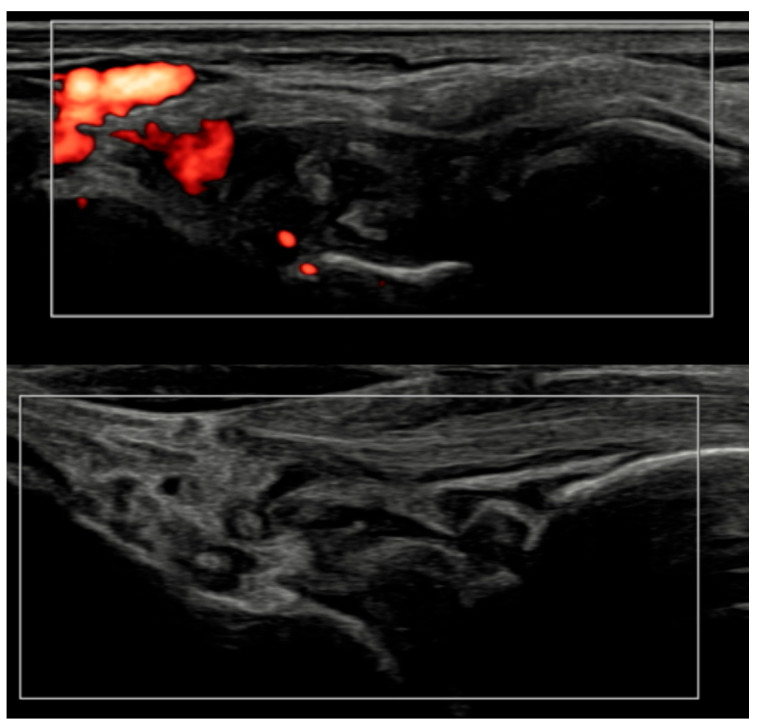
Longitudinal ultrasound comparison of the wrist joint at baseline (T0, top) showing synovial hypertrophy (grade 2) with intra-articular power Doppler signal (PD grade 2), corresponding to a combined EULAR–OMERACT synovitis grade = 2 (joint-level), and at 6 months (T6, bottom), demonstrating near-complete resolution of synovial hypertrophy and power Doppler signal, with a joint-level EULAR–OMERACT synovitis grade = 0.
